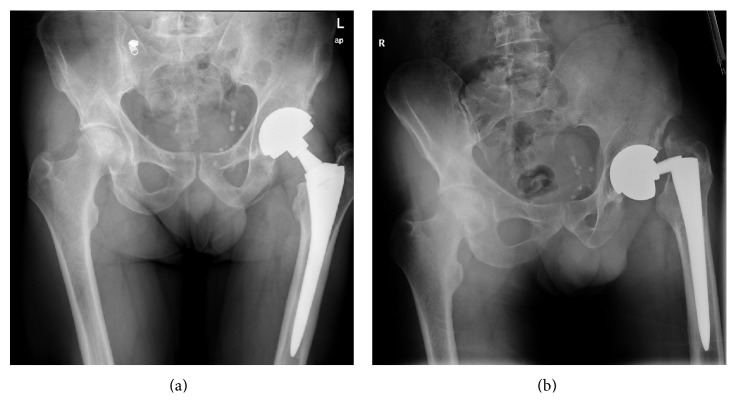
(a) Plains radiographs of the pelvis showing a Profemur Modular stem and Conserve Cup (Wright Medical Technology, Inc., 5677 Airline Road, Arlington, TN 38002) with a long modular titanium neck with 8 degrees of varus, metal on metal bearing surface, and a 50 mm big ball. (b) Emergency radiographs of the pelvis showing a rupture of the modular neck.
